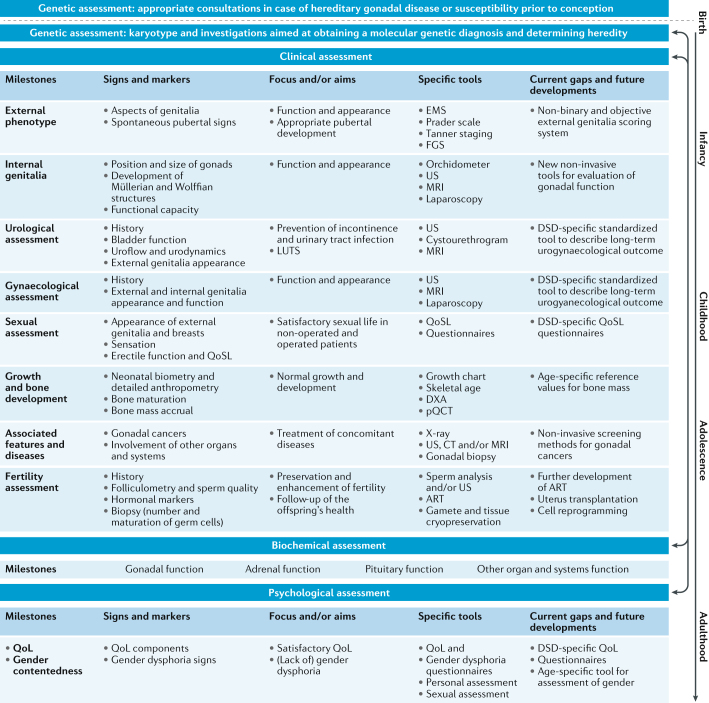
Assessment of a patient with a DSD in the clinical practice. Assessment of an individual with a (potential) difference of sex development (DSD) starts prenatally, followed by age-dependent relevant assessments throughout life. Holistic assessment includes genetic, clinical, biochemical and psychological investigations in a wide range of specific domains (left column). For each of these domains, defined signs and markers are available that can be used to screen for potential problems. The use of a pre-identified set of tools enables standardized assessment for each domain, focusing on relevant outcomes. Current gaps and future developments are listed in the right column. Dotted lines indicate inter-relations between the DSD patient and the family members and/or partners; solid lines indicate inter-relations between the different aspects of assessments according to the age of the DSD patient. ART, assisted reproductive technology; DXA, dual-energy X-ray absorptiometry; EMS, external masculinization score, FGS, Ferriman-Gallwey score; LUTS, lower urinary tract symptoms; pQCT, peripheral quantitative CT; QoL, quality of life; QoSL, quality of sexual life; US, ultrasonography.
